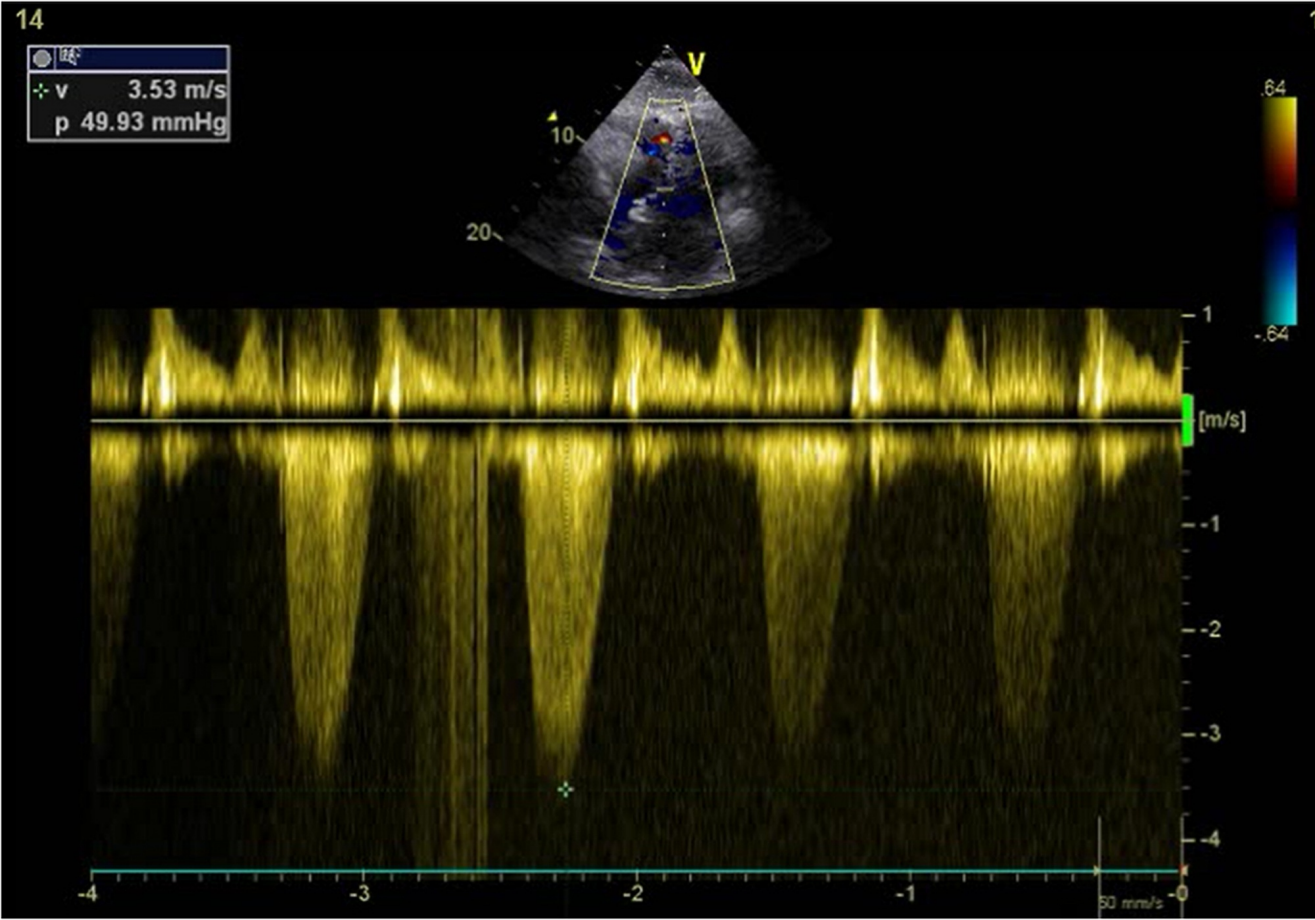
Echocardiogram. Continuous wave Doppler applied at the level of the pulmonic valve showing maximum pressure gradient of 50 mmHg denoting moderate pulmonary valve stenosis.
